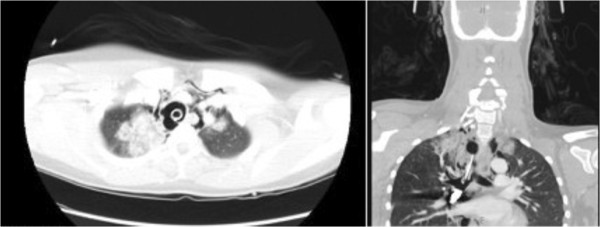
Computed tomography scan showing a posterior tracheal defect, and a bullet within the right bronchus.
